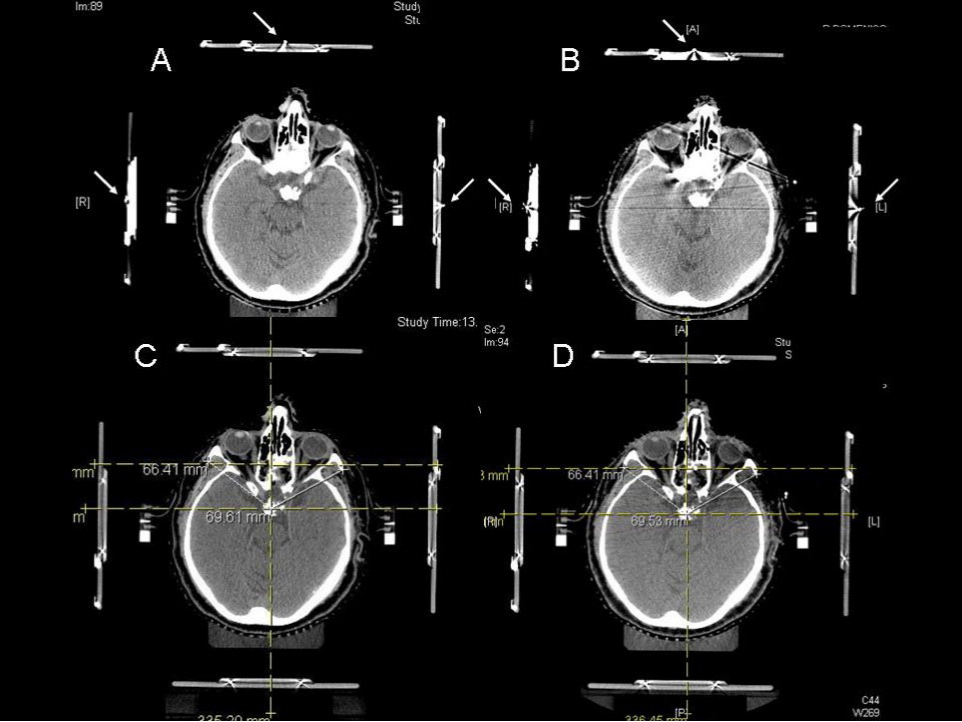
Verification of isocenter position accuracy. During CT localization (A) and CT verification (B) the patient is positioned on the CT couch with the target positioner box aligned with anterior and lateral lasers using the radio-opaque markers (arrows). The amount of isocenter shift between CT localization (planning CT) (C) and CT verification (D) in relation to anatomical skull base cranial structures was then evaluated directly on the CT scans.
